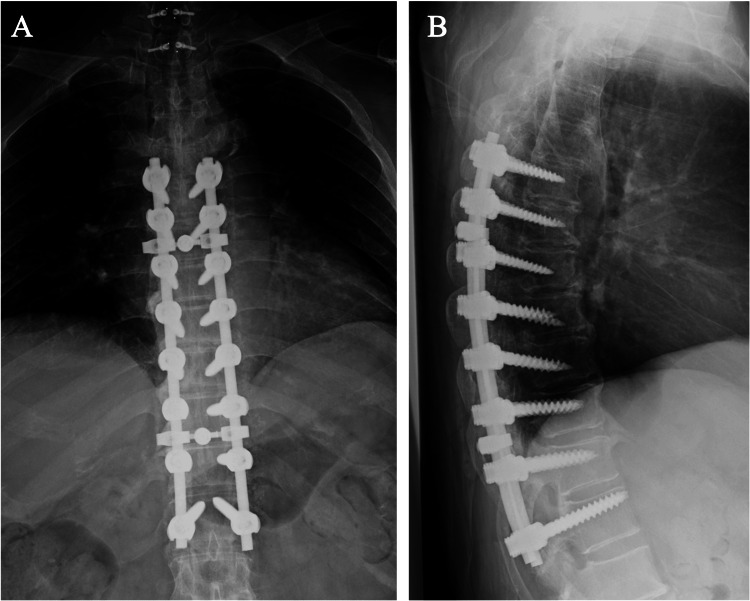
Postoperative radiograph of second surgery (Th5-Th12 decompression and fixation, A: anteroposterior, B: lateral)
